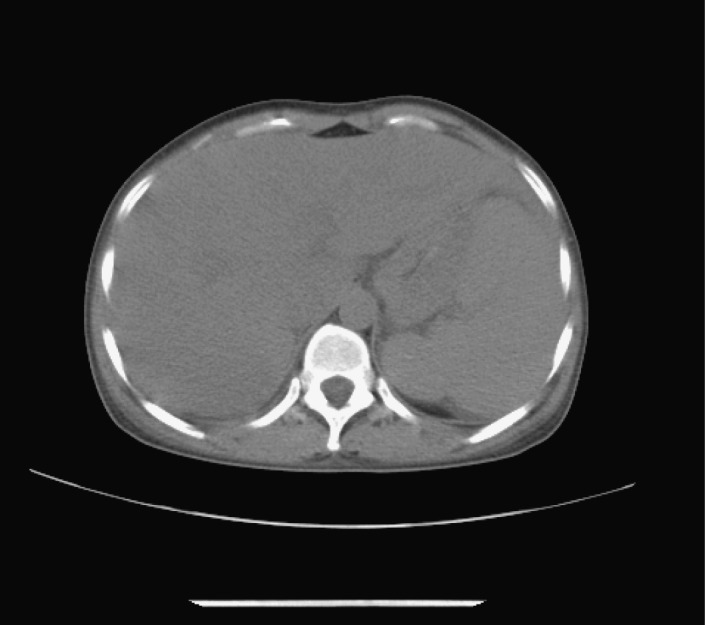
Abdominal computed tomography (CT). CT scan showed lower density of liver parenchyma and splenomegaly.
